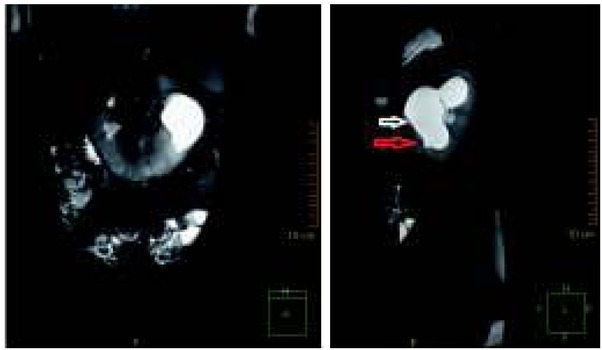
T2-zależne rekonstrukcje czołowa i strzałkowa urografii rezonansu magnetycznego nerki podkowiastej, z wodonerczem lewego układu i wysokim odejściem lewego moczowodu; biała strzałka – odejście moczowodu, czerwona strzałka – moczowód. Fig. 3. Coronal and sagittal T2-weighted magnetic resonance urography images of horseshoe kidney, with hydronephrosis of the left collecting system and high insertion of the ureter.
